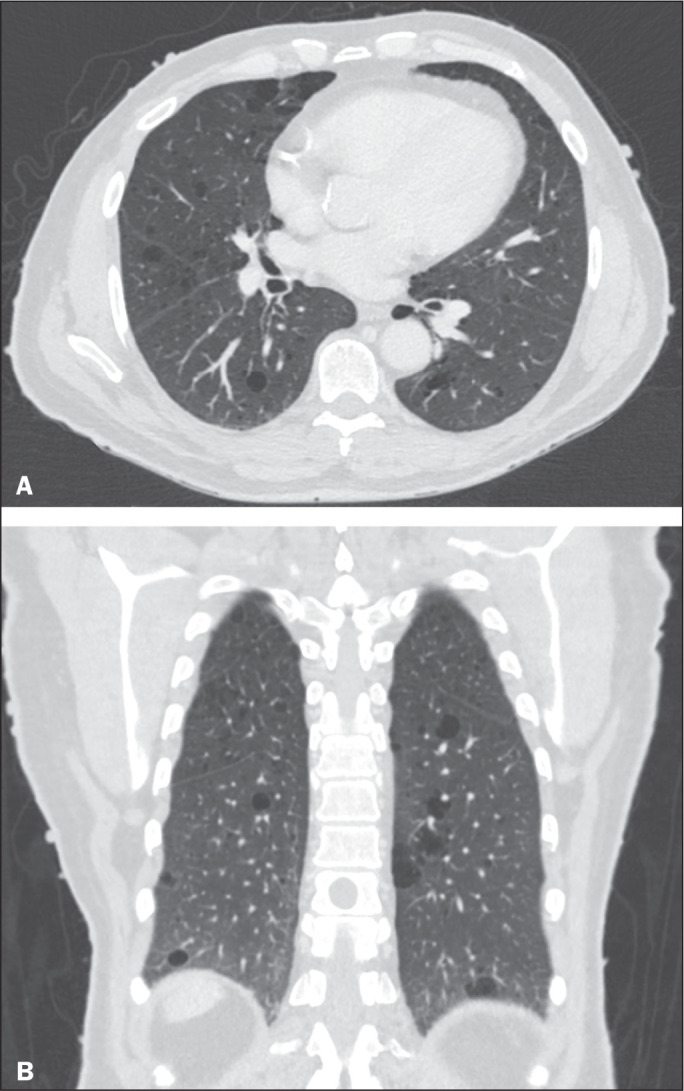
Chest CT of a 65-year-old man with NF1 and an unknown smoking history. Chest
